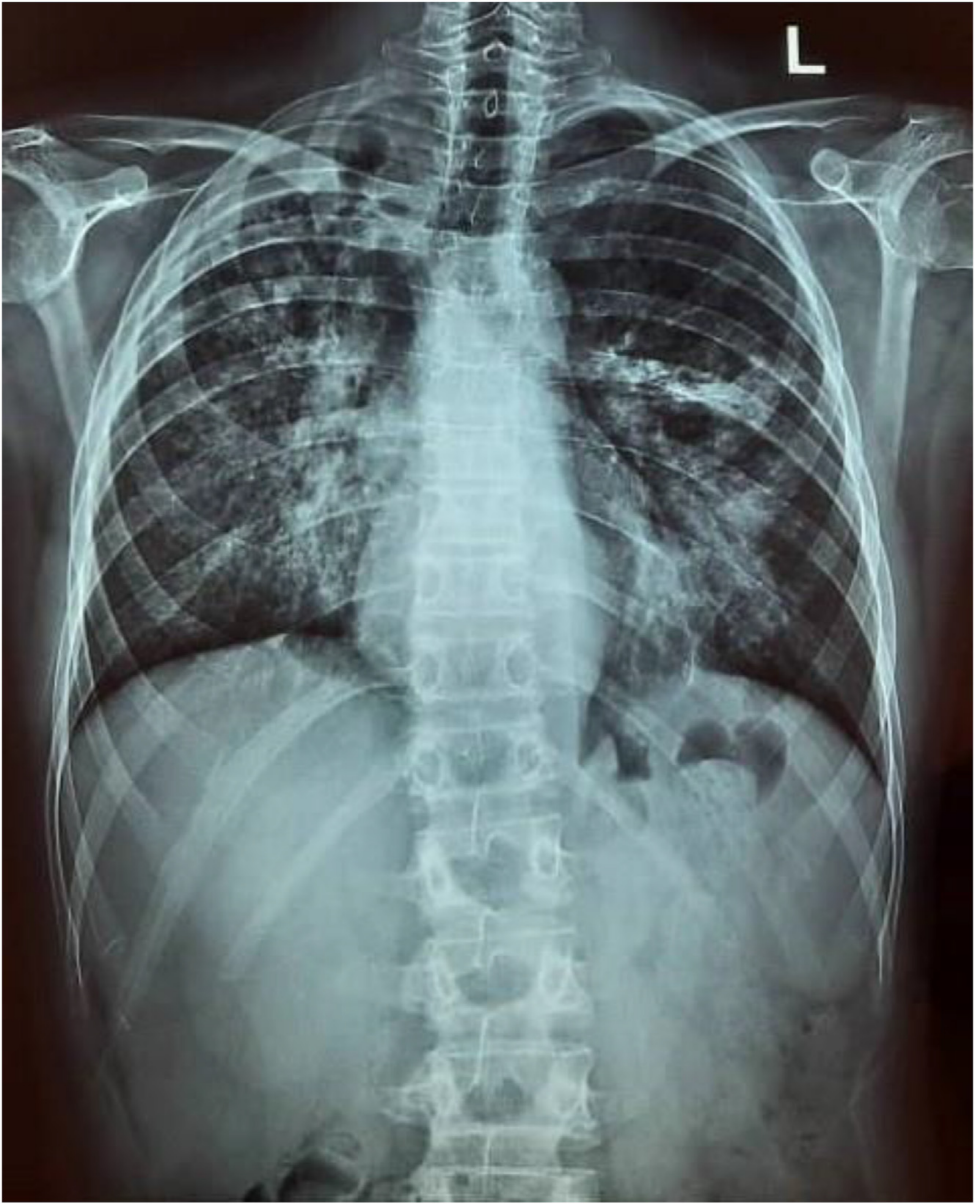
Chest X-Ray revealed Cavity with thick wall in right upper lobe along with the increase in thickness of pleura in the optic segment of the upper lob. Reticulonodular densities in the upper and middle zones of both sides and fibrosis, especially in the left middle zone, which are generally highly suggested for old TB.
